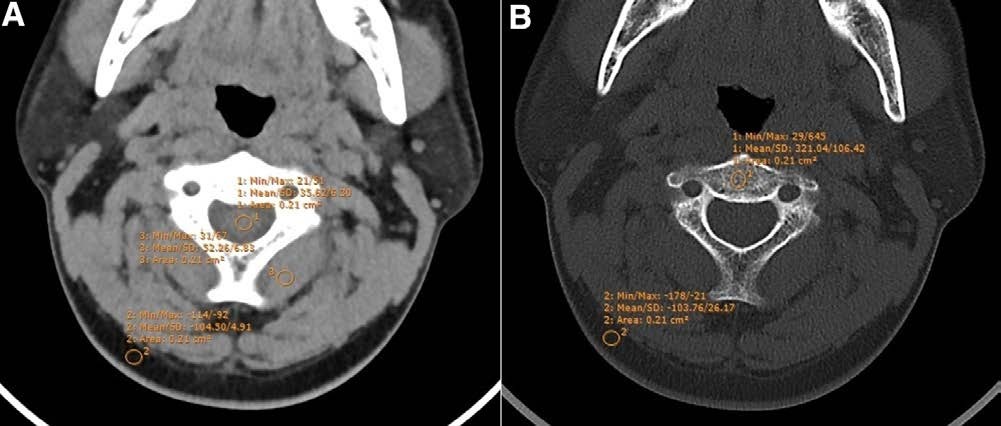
Example of ROIs measurement. The ROIs were placed at the dural sac (A 1), paraspinal muscles (A 3), subcutaneous fat (A 2, B 2), and cervical trabecular bone (B 1) (A: soft tissue window CT image; B: bone window CT image). CT = computed tomography, ROI = regions of interest.
